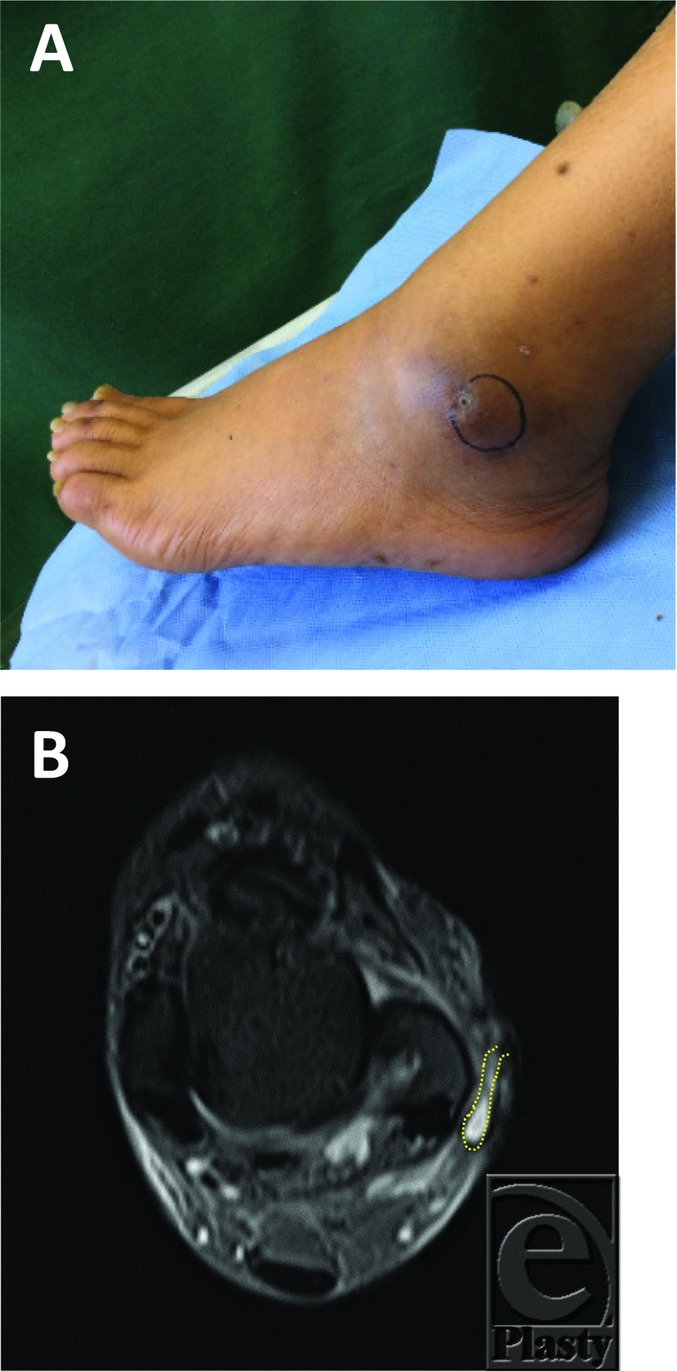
(a) Macroscopic finding showed that the pocket was reduced 10 weeks after the first PRP therapy. (b) Computed tomographic findings of the left lateral malleolus showed that the cavity size reduced after the first PRP session. The yellow dotted line indicates the margin of the subcutaneous cavity. PRP indicates platelet-rich plasma.
